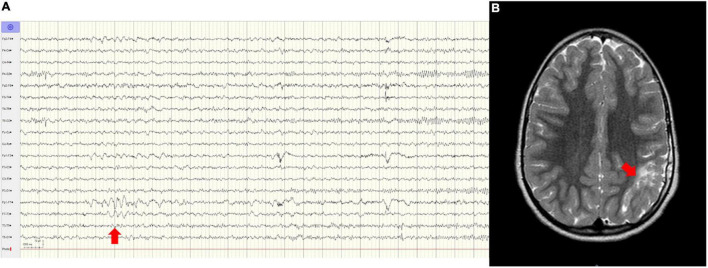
(A) EEG performed at the Emergency Department (ED) showing left fronto-temporal epileptiform abnormalities (arrow). (B) T2-weighted axial brain MR, showing the consequences of an old ischemic lesion in the left parietal lobe (arrow).
